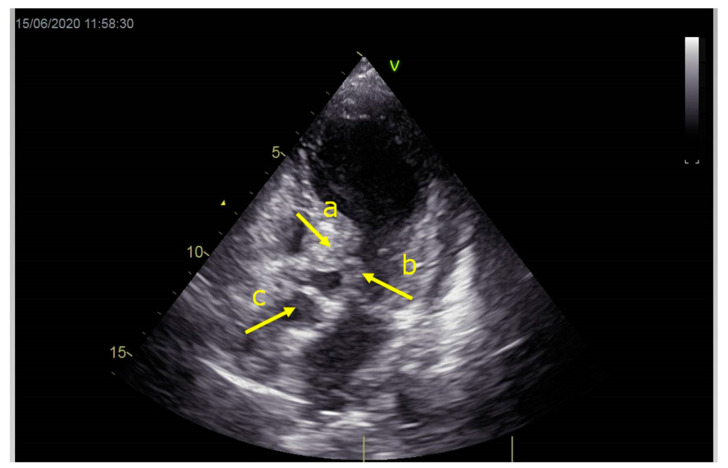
Echocardiography registered basal hyperkinetic segments of the left ventricle and formed a dynamic obstruction of the left ventricular outflow tract (a—LVOT, b—cuspi anterioris valvulae mitralis, c—aortic valve).
